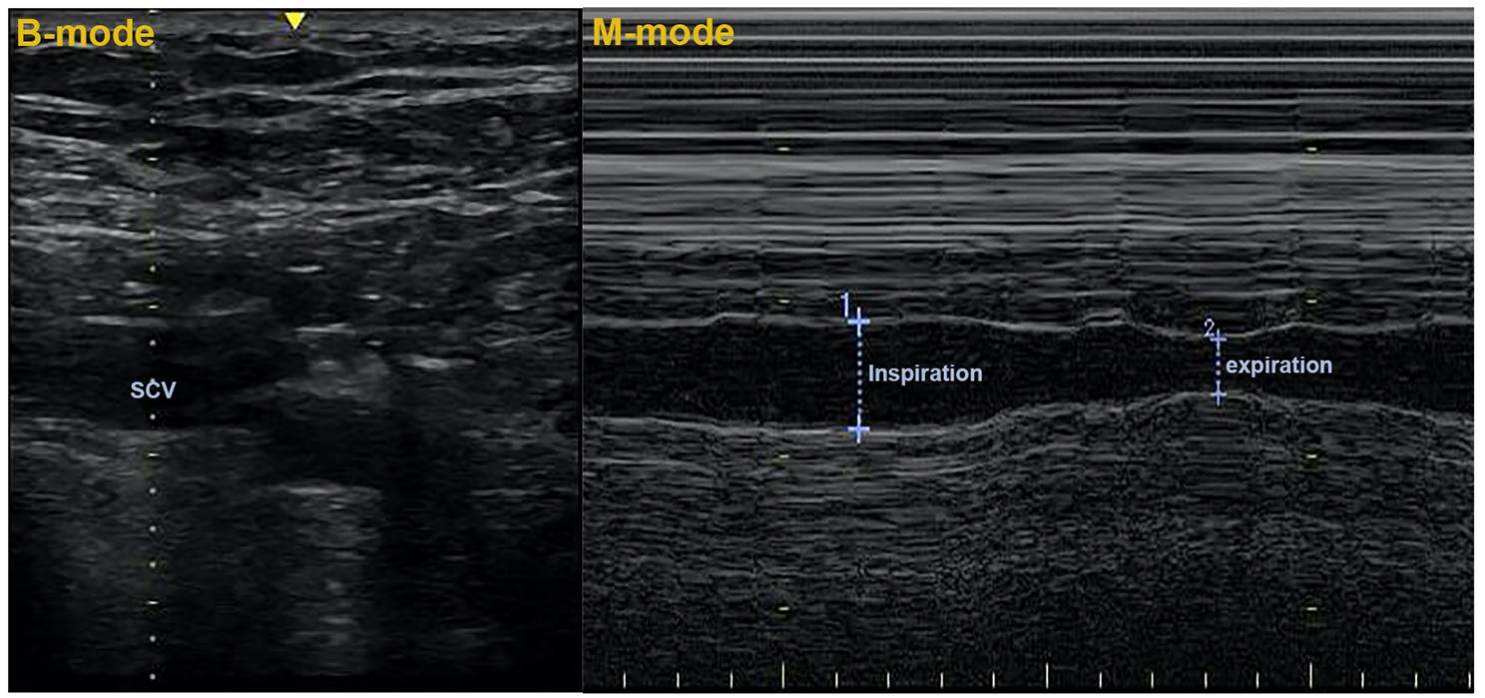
B-mode and M-mode ultrasonography to evaluate SCV with the subcostal view. The SCVCI was calculated as follows: SCVCI=(dSCVmax–dSCVmin)/dSCVmax×100%. The result therefore is expressed as percentages. SCV, subclavian vein; SCVCI, subclavian vein collapsibility index; dSCVmax and dSCVmin, the maximum and minimum antero-posterior diameters of the subclavian vein
